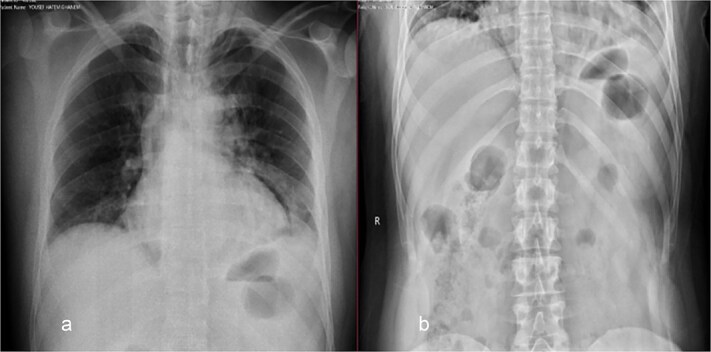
Chest (a) and standing abdomen (b) X-ray: Showed wide mediastinum with pneumomediastinum more at left side and right bronchus bulge. Normal standing abdomen X-ray.
